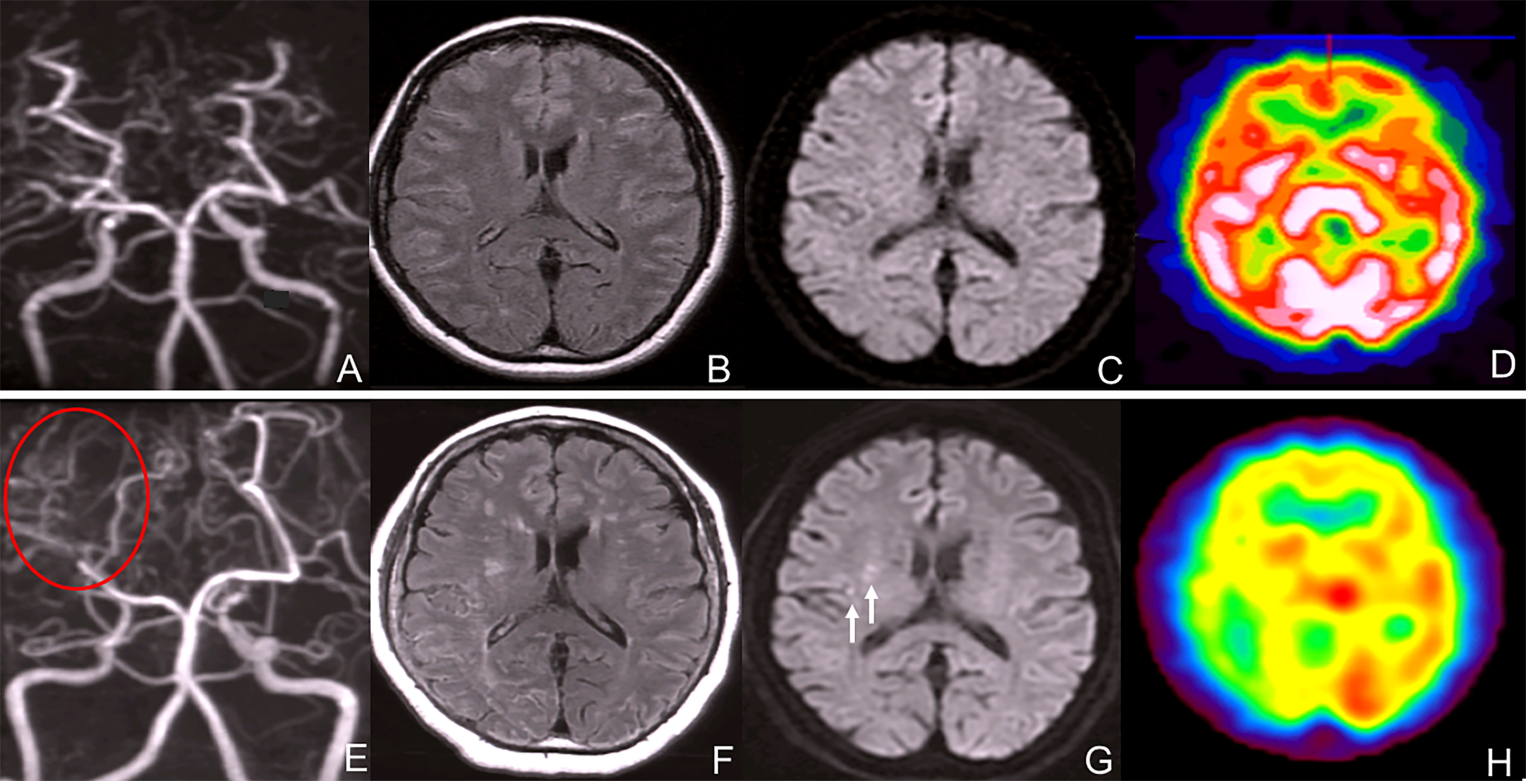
(A) Magnetic resonance angiography (MRA) revealed bilateral internal carotid artery stenosis. (B) MRI FLAIR showed no typical ischemic and hemorrhagic lesions in the brain. (C) MRI DWI showed no ischemic lesions. (D) SPECT showed no hypoperfusion or laterality of the blood flow. (E) Magnetic resonance angiography (MRA) revealed bilateral internal carotid artery stenosis and blood flow signal of PCA decreased. (F) MRI FLAIR showed bilateral ischemic lesions in the brain. (G) MRI DWI showed new ischemic lesions in the right deep white matter. (white arrows) (H) SPECT showed no hypoperfusion or laterality in the blood flow.
